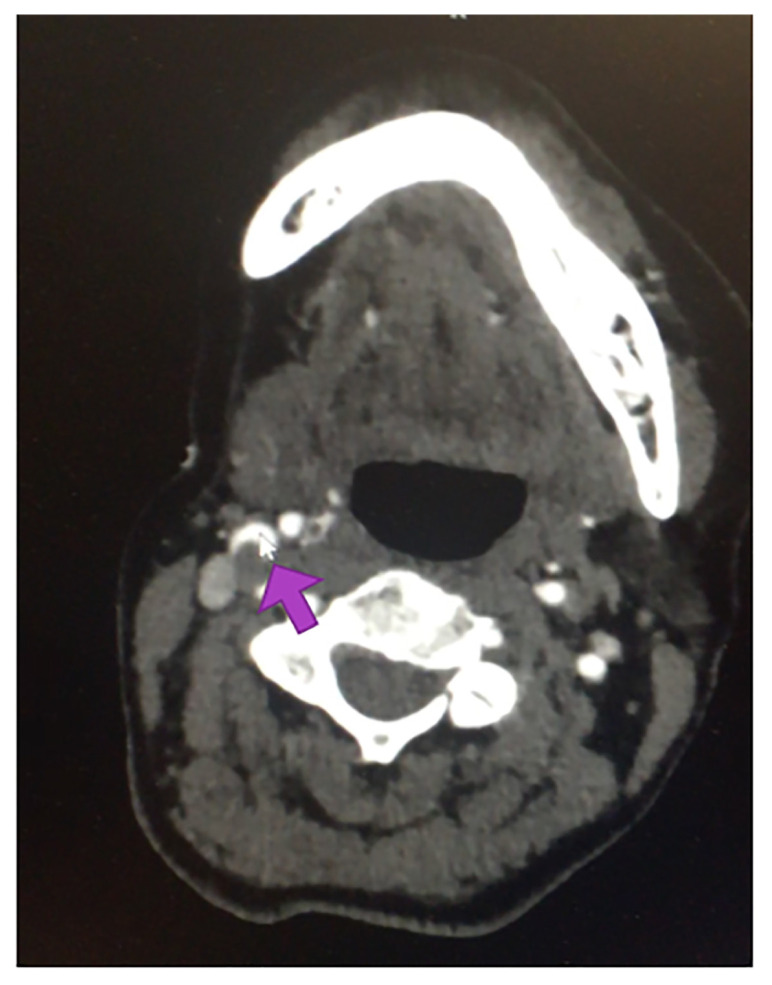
Computed tomography angiography of the neck identifying soft tissue at the right carotid bulb (arrow), mildly narrowing the lumen by less than 50%, potentially composed of soft atherosclerotic plaque with extensive thrombus.
